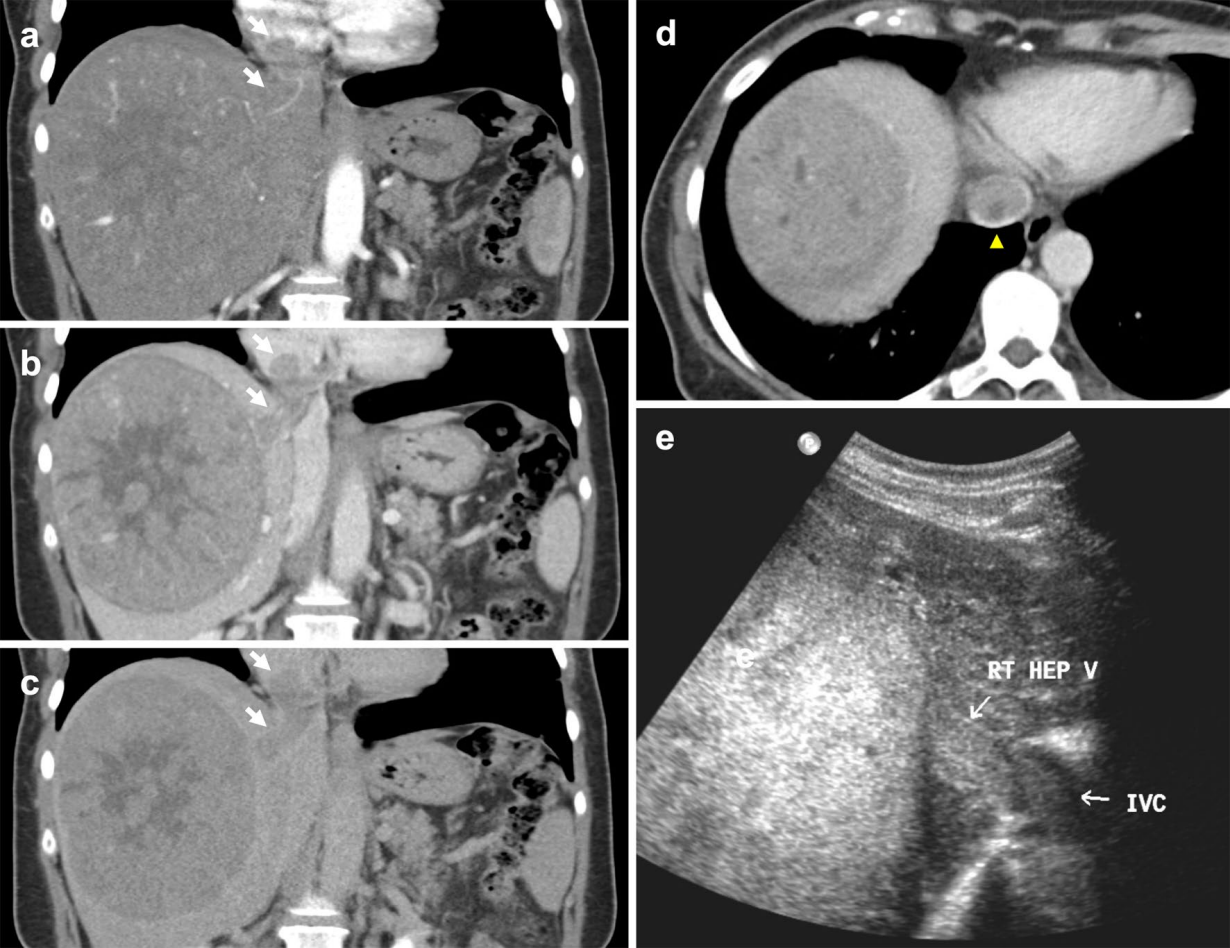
Hepatocellular carcinoma invading the IVC. a–c Coronal multiphase CT images show a 12 × 11 cm necrotic hepatic lesion invading the hepatic and supradiaphragmatic IVC. The thrombus (arrows) demonstrates enhancement in both (a) arterial and (b) portal venous phases, with complete washout in the (c) delayed phase. d Axial CT shows near complete obstruction of the hepatic IVC (arrowhead). e US Abdomen demonstrates a hyperechoic hepatic mass extending into the right hepatic vein and IVC
